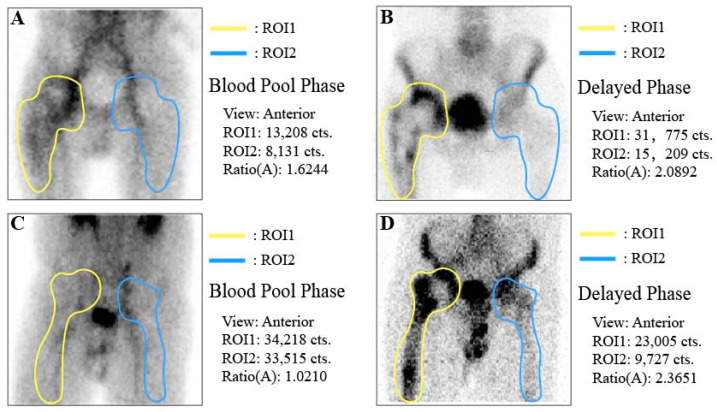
Bone scintigraphy of patients with PJI and aseptic loosening. (A,B) Male, 67 years old, diagnosed with right hip PJI; (C,D) Male, 72 years old, with right hip aseptic loosening. ROI: region of interest.
